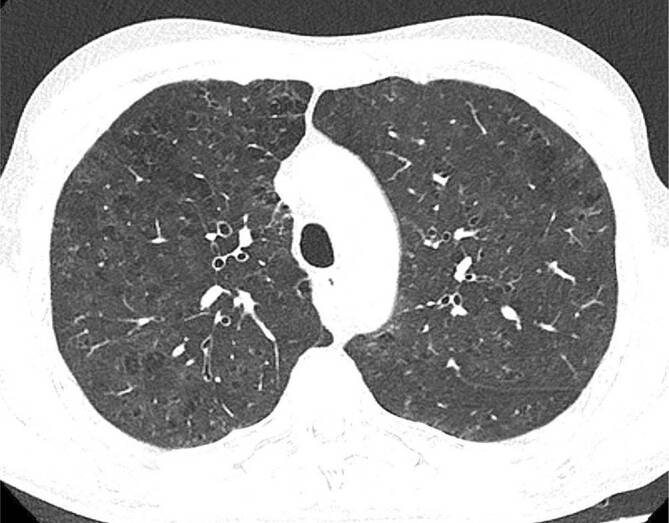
Die RB-ILD wird zunehmend ohne Lungenbiopsie diagnostiziert, wenn die typischen CT-Veränderungen sowie pigmentierte Makrophagen in der bronchoalveolären Lavage vorliegen und keine Lymphozytose besteht. Diese würde eher für eine EAA sprechen, die bei Raucher*innen sehr selten ist [16, 17].
